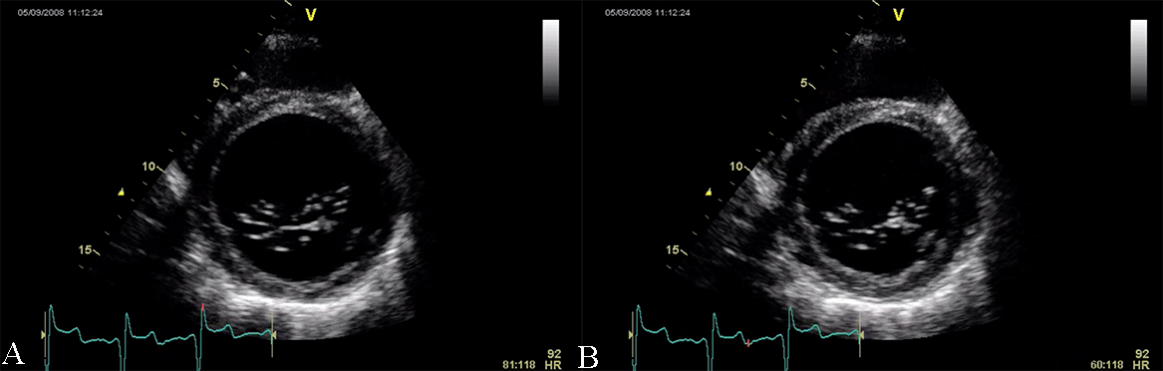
Echocardiographic aspect of DCM in a patient with idiopathic
